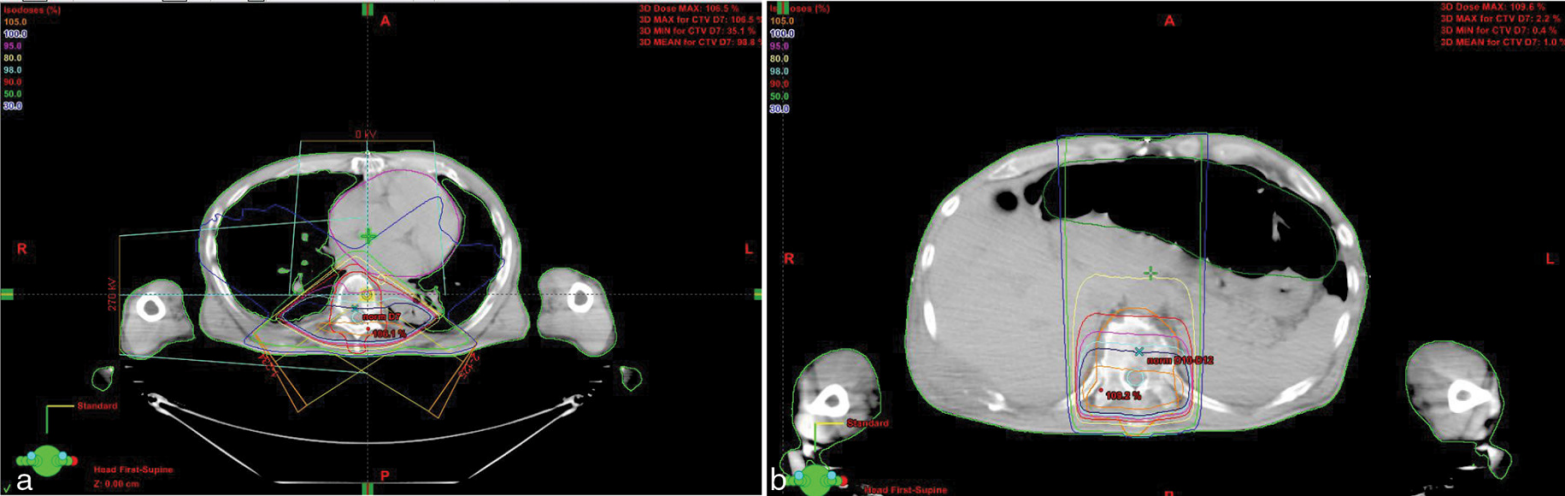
The CT-based plan of three-dimensional conformal radiotherapy using a linear accelerator with 18 MV photon beam. The treatment of T7 was performed using two oblique posterior–anterior fields (a) while the treatment for T10–T12 was performed using a direct posteroanterior field (b). The target volume was contoured by the red line in (a) and orange line in (b). The red and yellow isodoses encompass the area covered by 90% and 80% of the prescribed dose, respectively.
